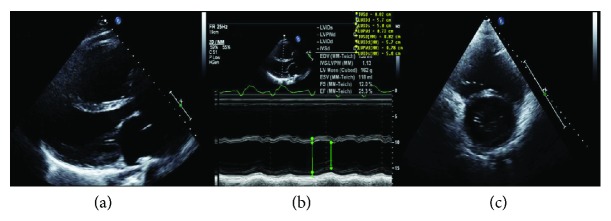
Echocardiogram: (a) parasternal long axis view showing right and left ventricular dilatation, (b) M-mode with left ventricular measurements, and (c) parasternal short axis view at mitral valve level with biventricular enlargement.
